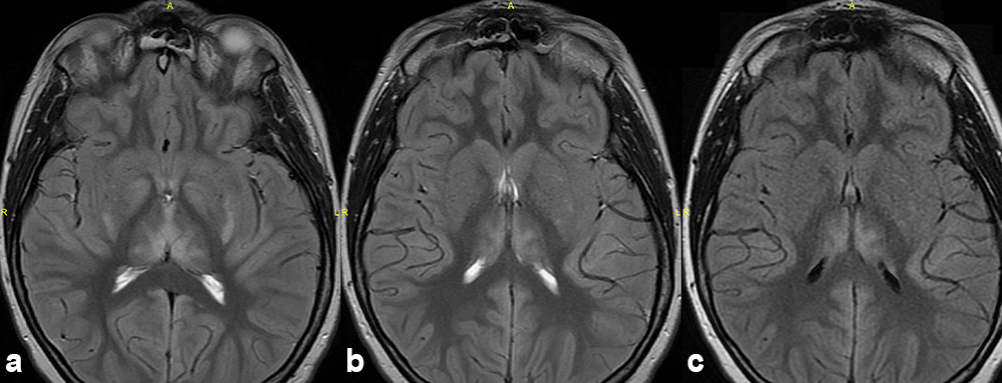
MRI brain images of the same patient in Figure 1. Axial *T*_2_ weighted images (a, b) demonstrate symmetrical hyperintensity in the thalami and basal ganglia regions. Axial FLAIR (c) MRI image shows no hyperintensity involving the internal capsule, and the head of the caudate nucleus is not atrophied. FLAIR, fluid attenuation inversion recovery.
